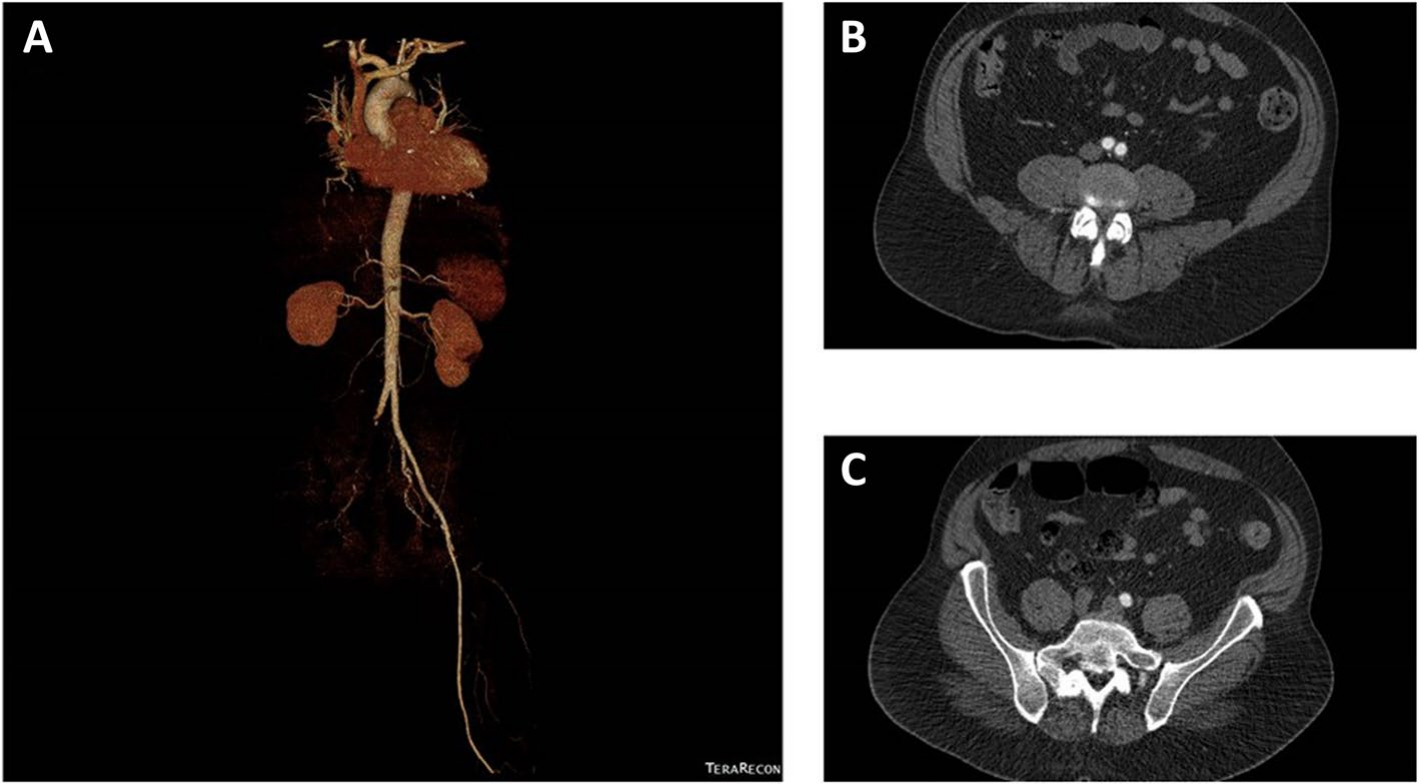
Three dimensional Volume Rendering Technique (VRT) of the computed tomography angiogram demonstrating A right-sided pelvic occlusion, and B axial computed tomography scan demonstrating both pelvic arteries and C only the left-sided arteria iliaca communis and right-sided occlusion of the arteria iliaca communis
